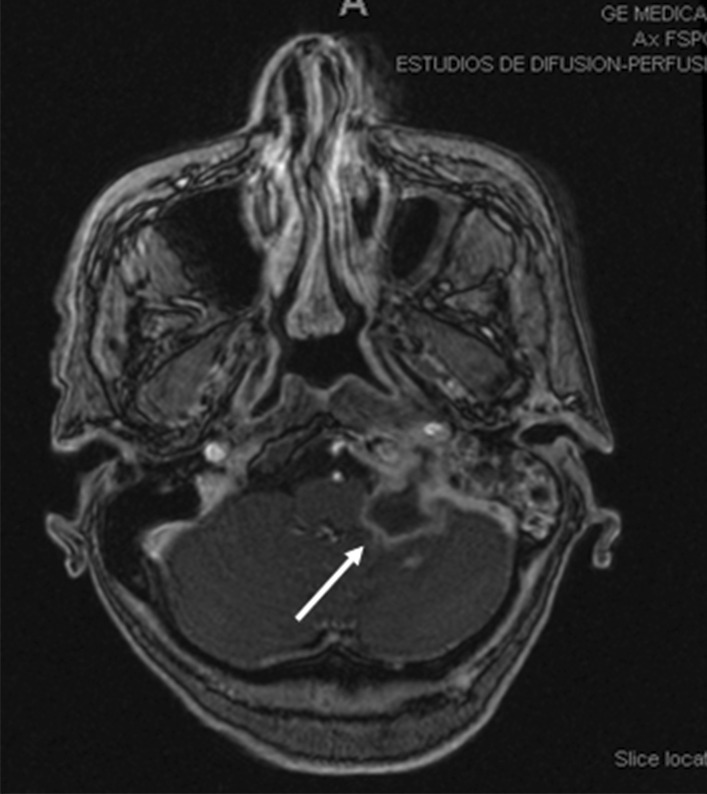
Magnetic resonance imaging October 2018 showing a slight decrease of the metastatic lesion at the base of the skull
